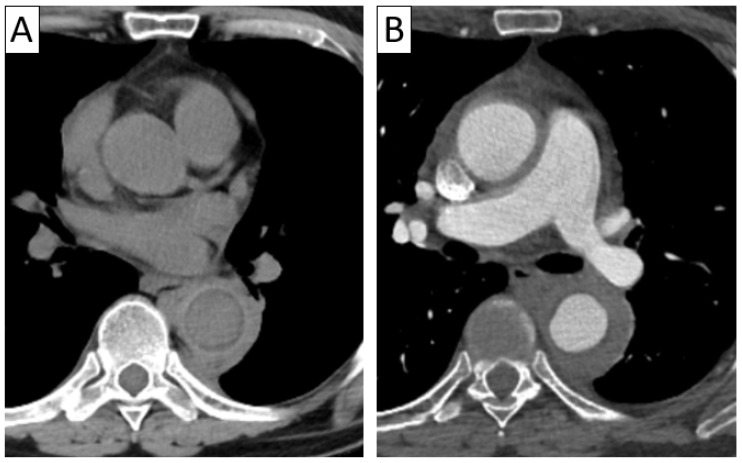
Contrast-enhanced axial thoracic CTA in a patient with TAK showing a highly attenuating thickened wall of the descending aorta on unenhanced scan (A) and the “double ring” sign on venous-phase imaging (B): a hypodense internal ring (intima) surrounded by an enhancing outer ring (media and adventitia).
